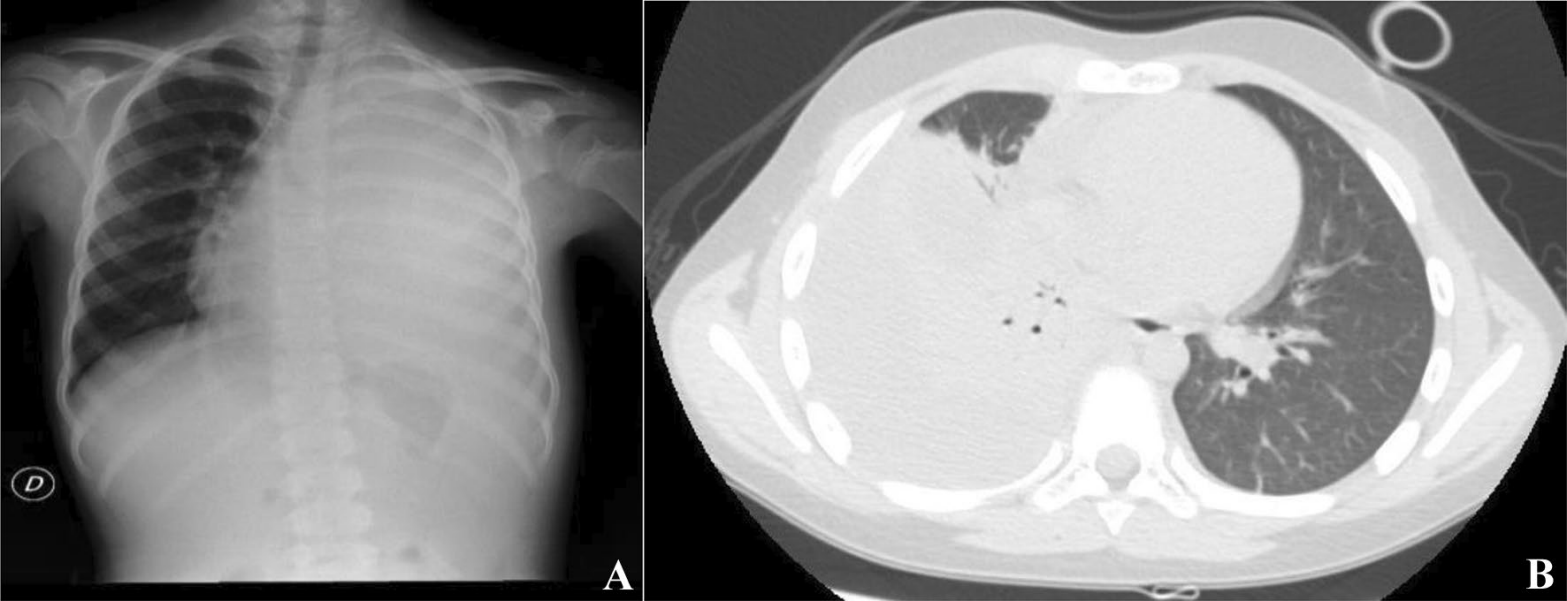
a Chest XR of left pleural empyema. b CT scan of right pleural empyema
